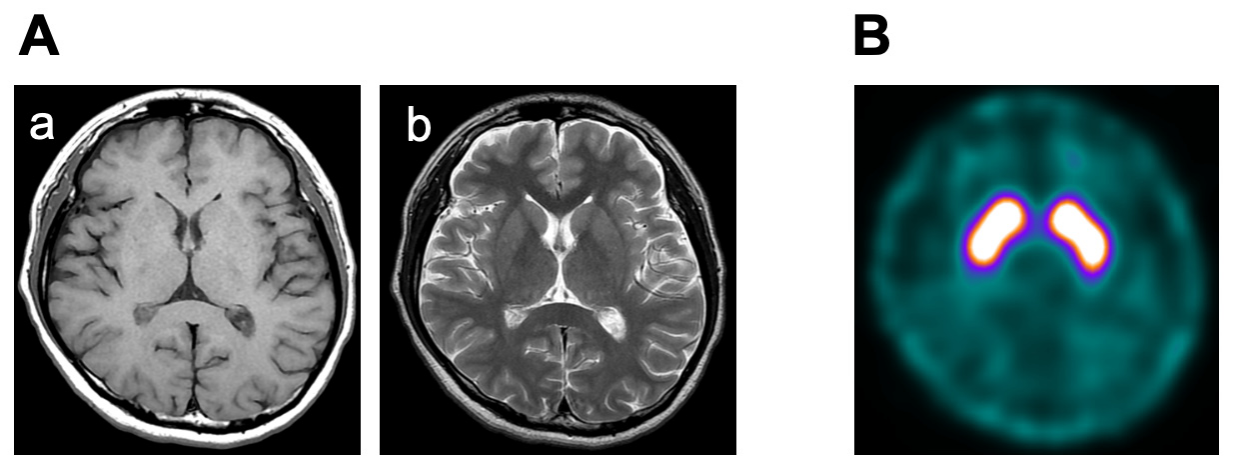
A: Cranial magnetic resonance imaging shows no abnormality in (a) T1 and (b) T2 axial sequences.
